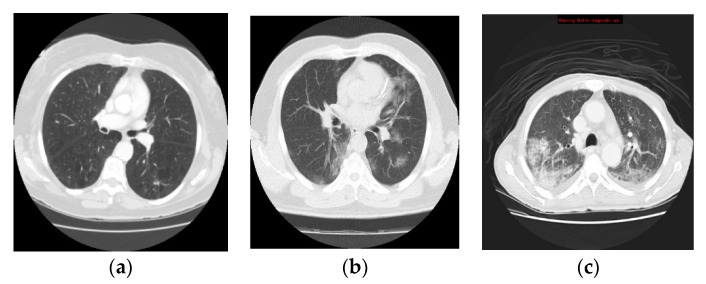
CT scans of the lung from the collected dataset: (a) healthy lung, (b) COVID-19 infection and (c) pneumonia infection.
